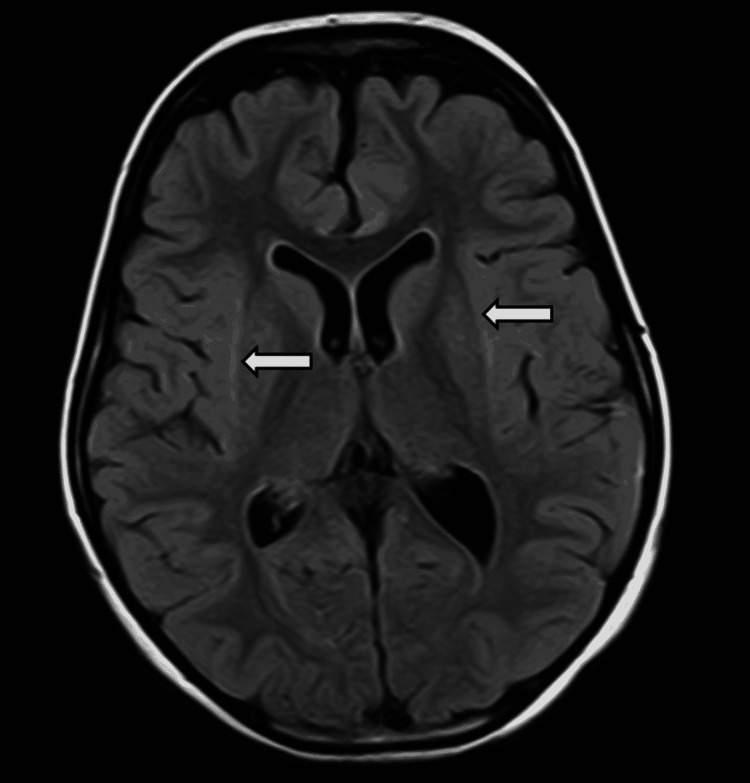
Magnetic resonance imaging of the brain (fluid-attenuated inversion recovery) White arrows pointing to the hyperintense claustrum.
